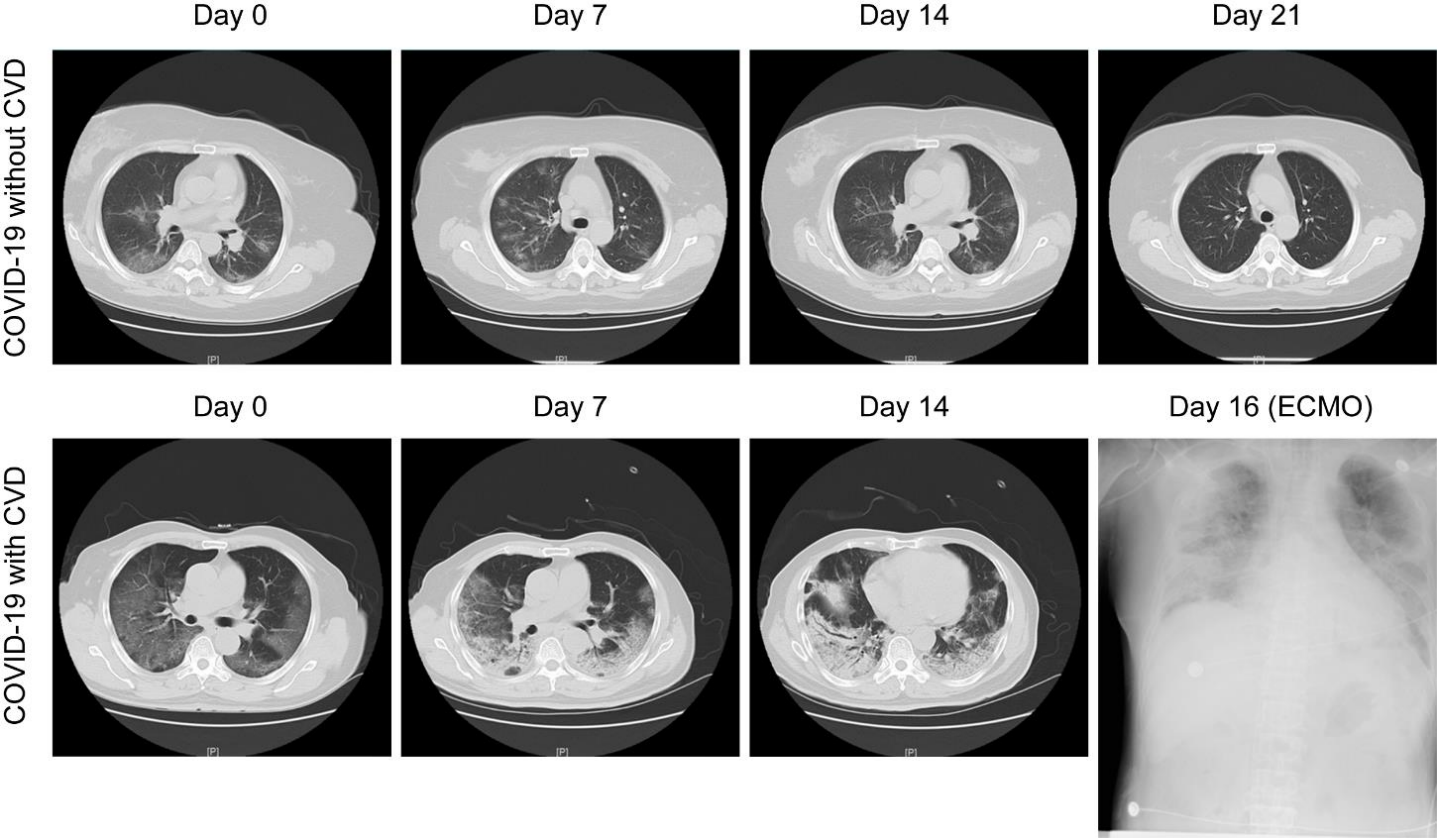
Representative chest computed tomography (CT) images of COVID-19 pneumonia in a non-cardiovascular disease (CVD) case and a CVD case. Top panel: A 60-year-old man with COVID-19, but not CVD: chest CT images showed ground-glass opacity (GGO) and patchy consolidation with peripheral and subpleural distribution, which had been absorbed at 21 days after hospitalization with treatment. Bottom panel: A 65-year-old man with both COVID19 and CVD: chest CT images showed diffusely subpleural consolidation with a crazy-paving pattern. Diffuse shadowing and consolidation were seen on chest radiography after intensive care unit (ICU) admission with extracorporeal membrane oxygenation (ECMO) support at day 16.
